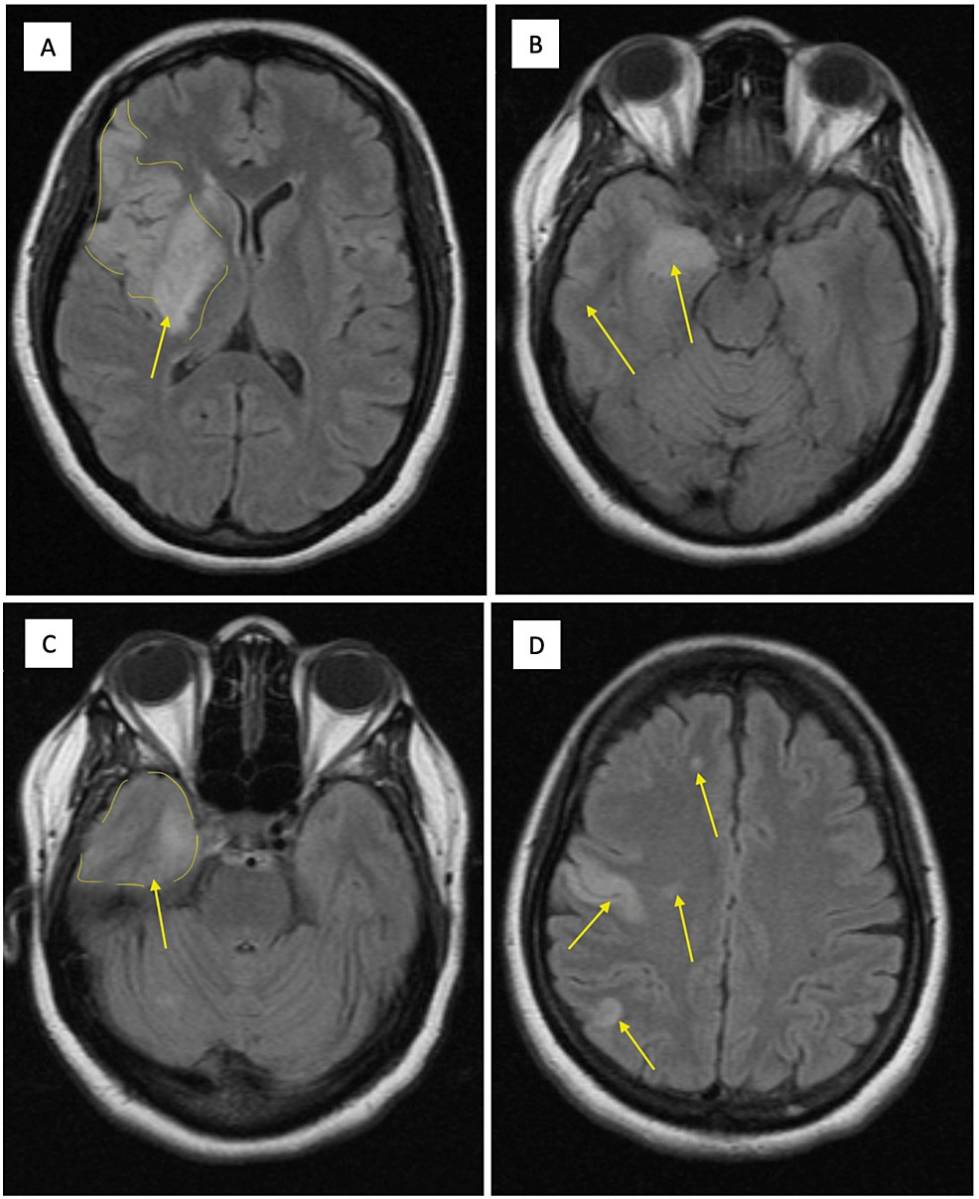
Magnetic resonance imaging Magnetic resonance imaging showing an ischemic stroke on the right side of the brain is shown in yellow arrows (A, B, C, D).
